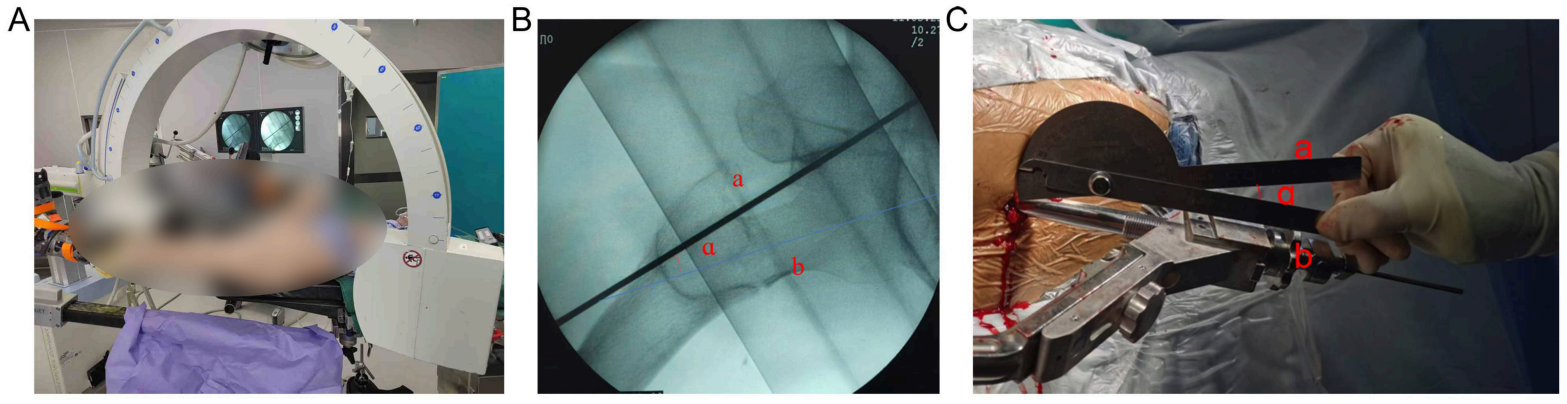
(A) Rotate the C-arm to achieve a true lateral view of the femoral neck. This position is confirmed when the guide wire, as visualized on the X-ray receiver, is parallel to the ground. (B) On the lateral view of the femoral neck, the following parameters are defined: Line a: the positioning Kirschner wire, which serves as the horizontal reference line; Line b: the central axis of the femoral neck; Angle ɑ: the angle formed between Line a and Line b, referred to as the horizontal anteversion angle. This angle represents the relationship between the central axis of the femoral neck and the horizontal plane. (C) The horizontal anteversion angle (ɑ) is measured using an angle measuring ruler. The horizontal arm of the ruler is aligned parallel to Line a, and the movable arm is aligned with Line b. The angle displayed between the two arms represents the horizontal anteversion angle.
